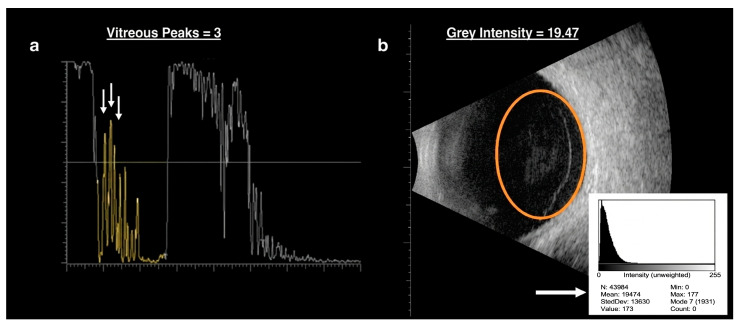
Methods of A-scan (a) and B-scan (b) analysis. (a) Example of an A-scan ultrasound image showing vitreous echoes. Significant vitreous peaks (white arrow) were defined as those exceeding 50% of the vertical axis and were manually counted. (b) Example of a B-scan image with superimposed ellipsoidal Region of Interest (ROI, 200 × 280 pixels, orange circle) placed in the posterior vitreous cavity. Gain settings were fixed at 110 dB for all B-scans.
